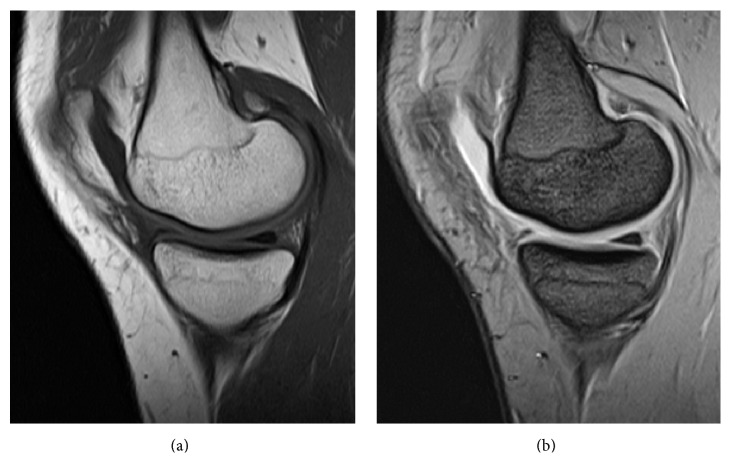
No abnormal signals adjacent to the anterior horn of the medial meniscus were noted on magnetic resonance images at 12 months postoperatively.
